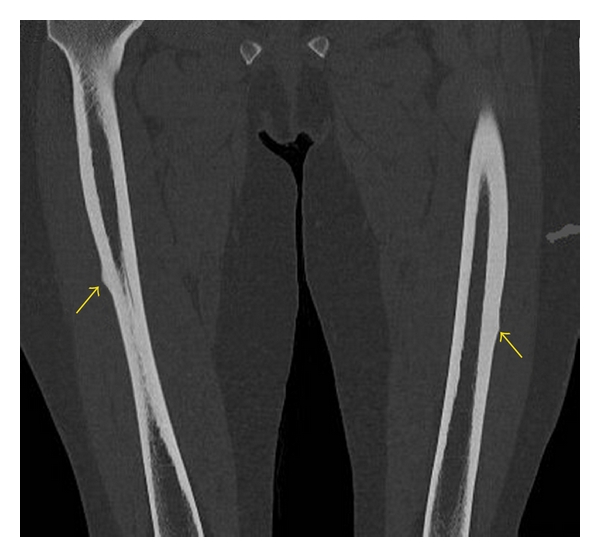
Computed tomography reveals bilateral femoral stress fractures.
